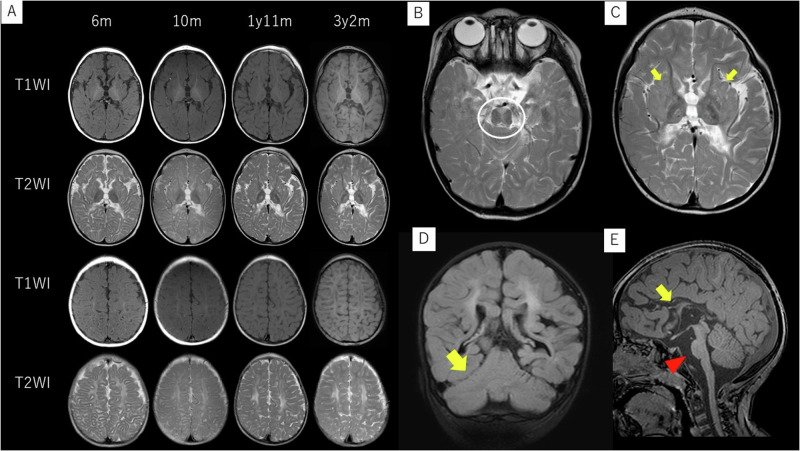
Brain MRIs from this case. A Axial T1-weighted and T2-weighted images are shown alternately. From left to right, images were obtained at 6 months, 10 months, 1 year and 11 months, and 3 years and 2 months of age. Myelination is severe delayed but shows slow progression over time. B, C Axial T2-weighted images show characteristic “figure of 8” midbrain appearance (white ovals) and small basal ganglia (yellow arrows). D Coronal T2-FLAIR images reveal hypoplasia/atrophy of the cerebellar hemispheres with relative sparing of the vermis (yellow arrows). E Sagittal T1-weighted images show small pons (red arrowheads) and extreme thinning of corpus callosum (yellow arrows). MRI magnetic resonance imaging.
